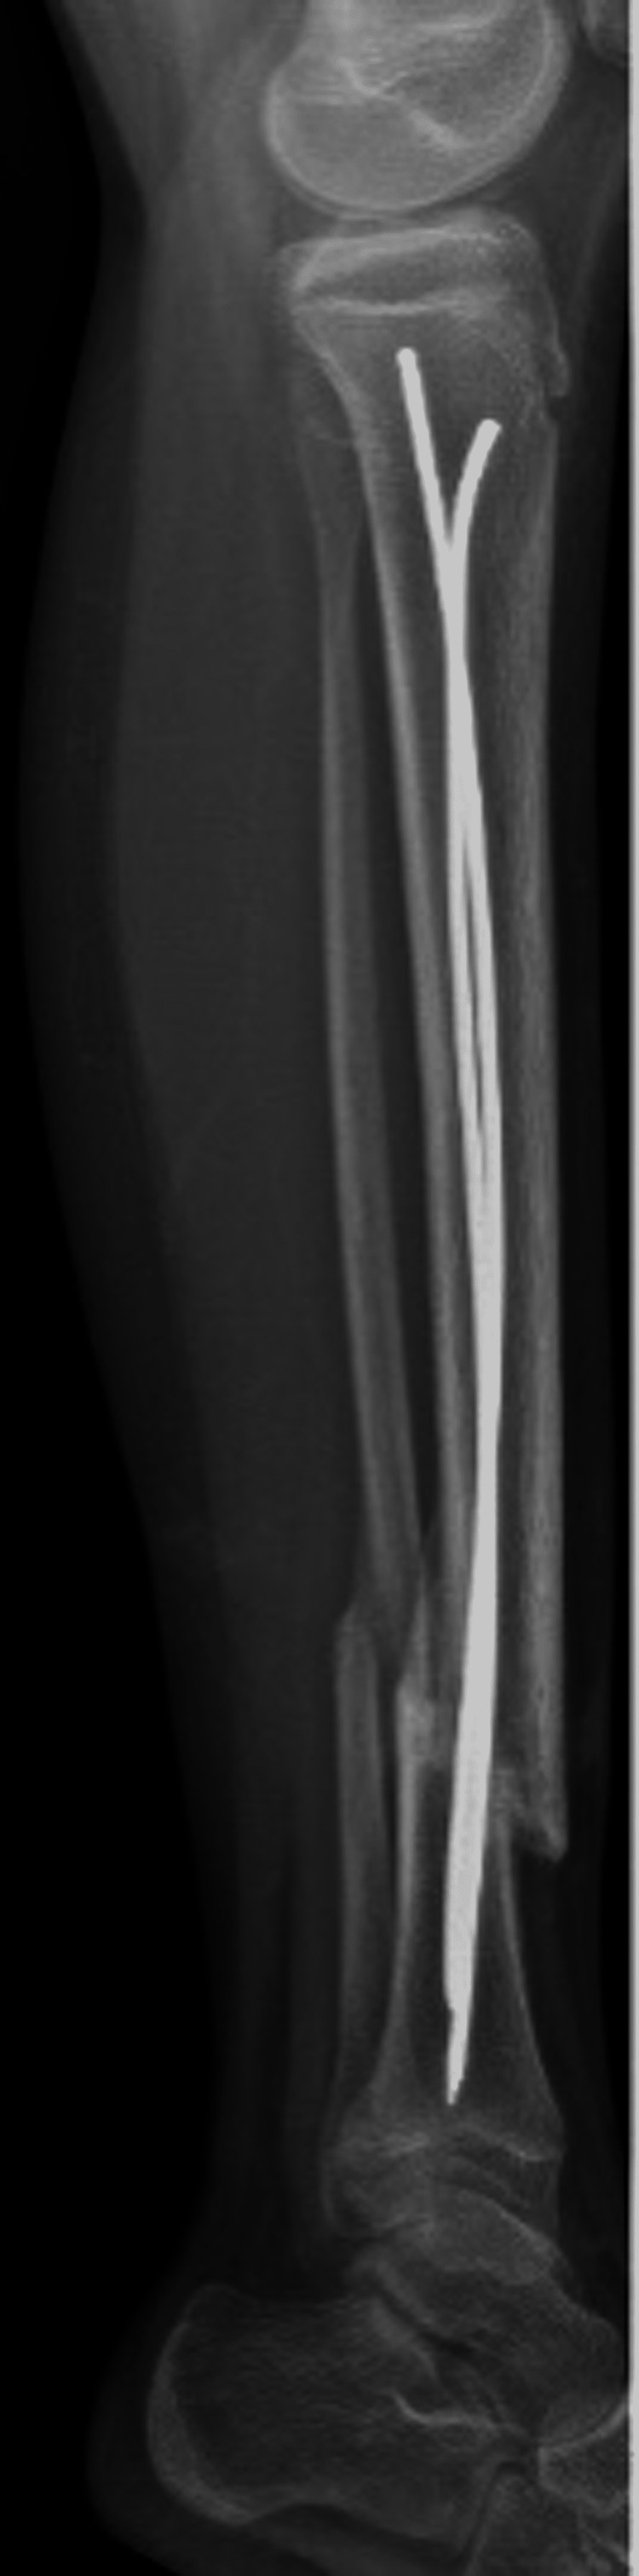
Three-month post-operative lateral tibia-fibula radiographs of flexible nailing of tibial shaft.
